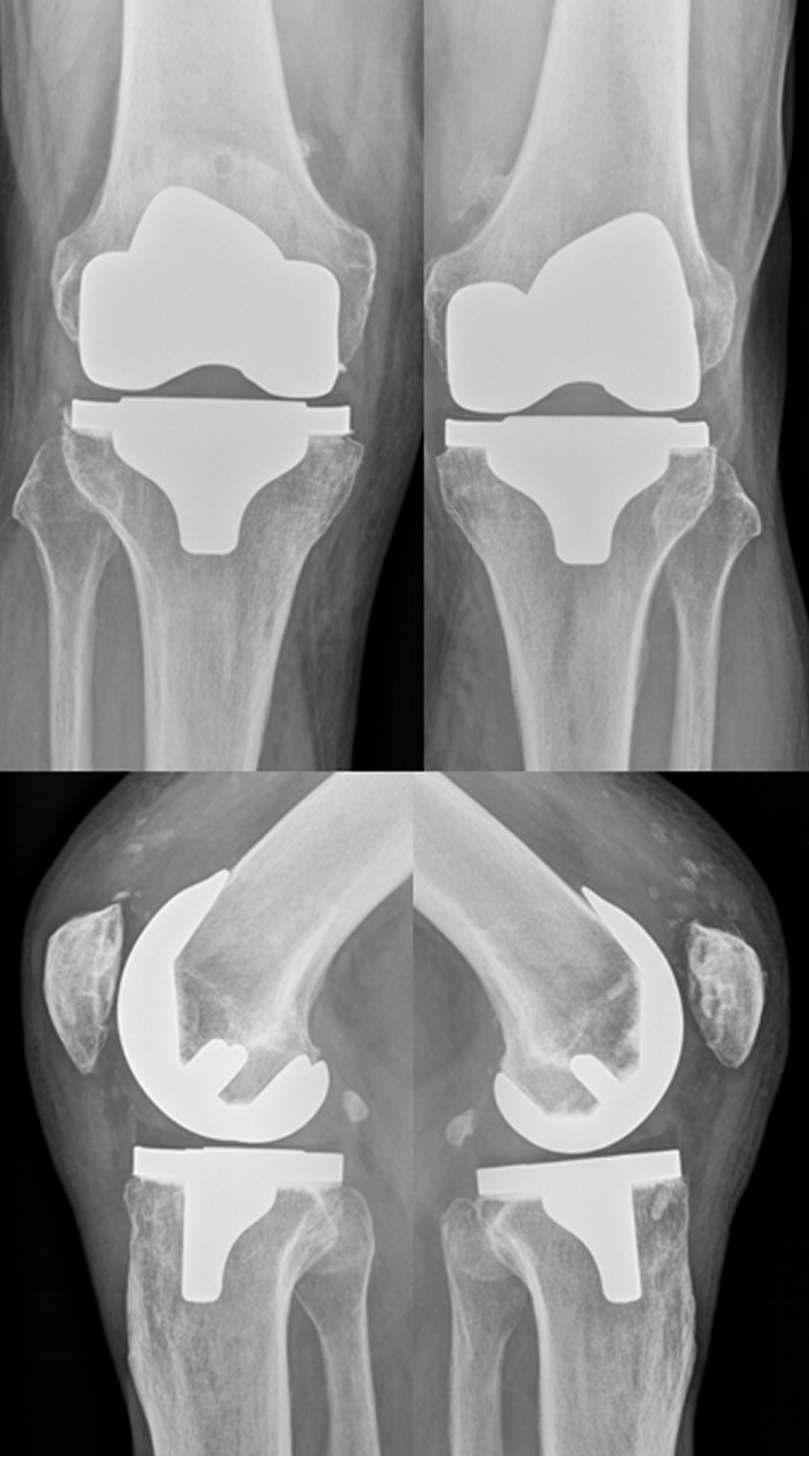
Radiographs ap and lateral of the knees 25 months after surgery. The picture shows correctly implanted bilateral TKAs.
